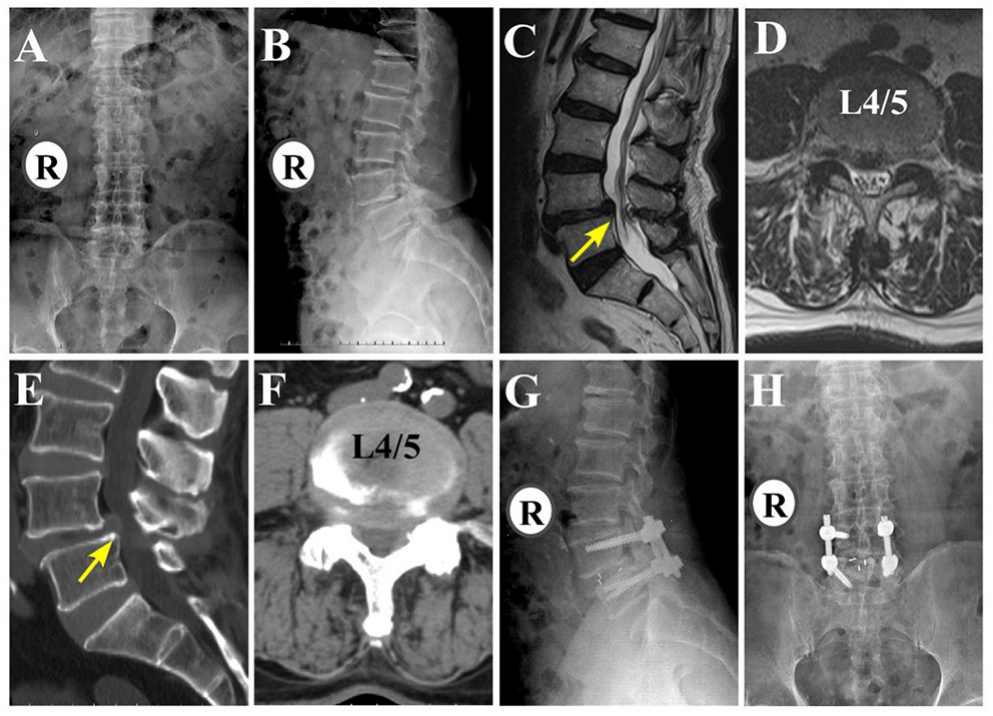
An 80-year-old man with low back pain and left lower extremity radiculopathy with MIS-TLIF (L4/5). (A,B) Preoperative anteroposterior and lateral fluoroscopy. (C,D) Preoperative sagittal and axial MRI (E,F) Pre-operative sagittal and axial CT. (G,H) Postoperative anteroposterior and lateral fluoroscopy.
